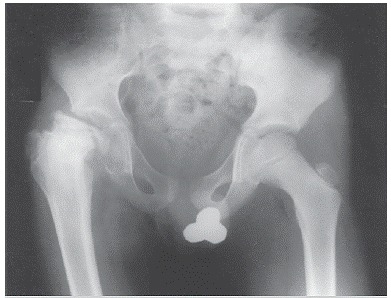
Anterior posterior radiograph of the pelvis, ato ne year evolution after trauma, showing a major colapse and subluxation of the femoral head to the right, characteristic of osteonecrosis of the femoral head.
